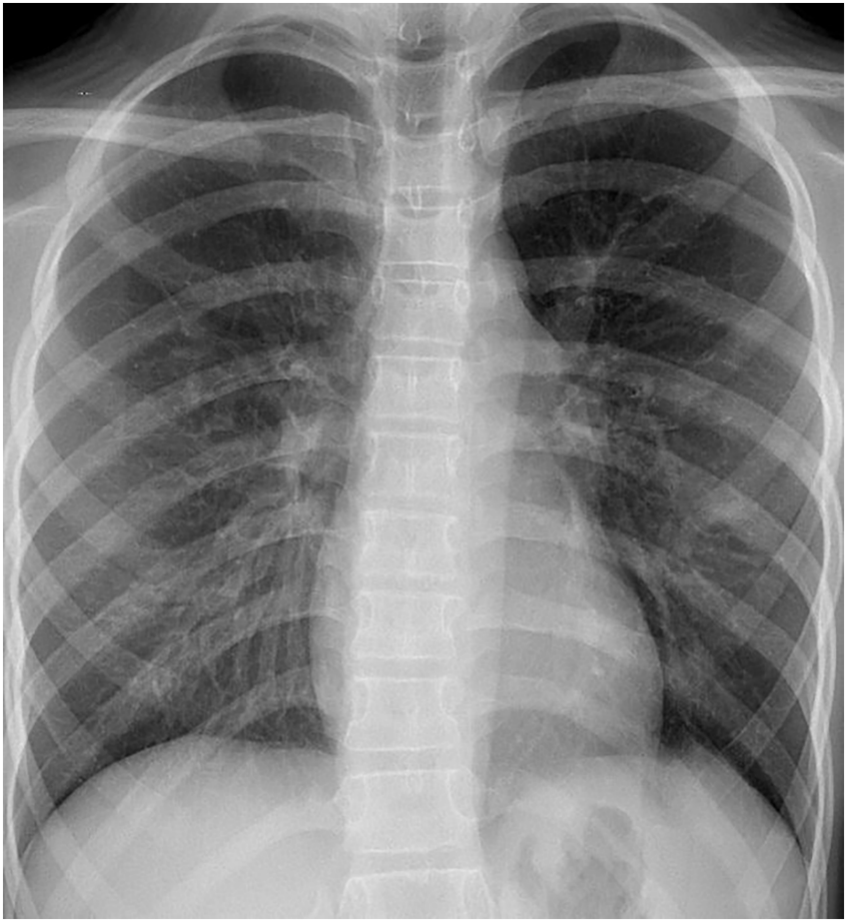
Chest plain radiograph of the child at the time of admission; no basis for pulmonary arteriovenous fistula was found.
